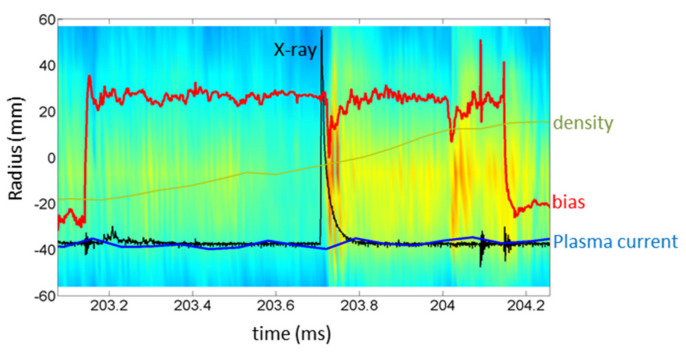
The colored background represents the contour plot of ‘*n sigma*’ evolution (a.u.) covering the plasma radius from about ±50 mm. Superimposed signals show the plasma current, plasma density and electrode biasing voltage (a.u.). It is clearly seen that, following the X-ray signal, the ‘*n sigma*’ profile increases in the plasma core region.
